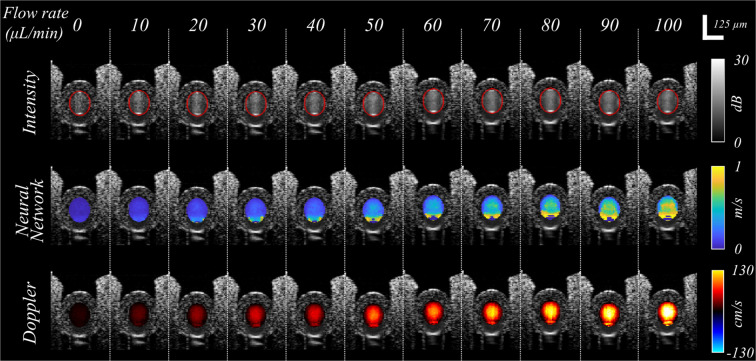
The NN and Doppler OCT measurements with varying flow rate. The first row shows intensity images of the structures of the tubing. The second row shows the NN velocimetry data from inside the tubing as an overlay on the intensity images. The third row shows the Doppler OCT velocimetry data as an overlay on the intensity images. Each column as denoted by the white dashed lines represents a different tubing infusion flow rate.
